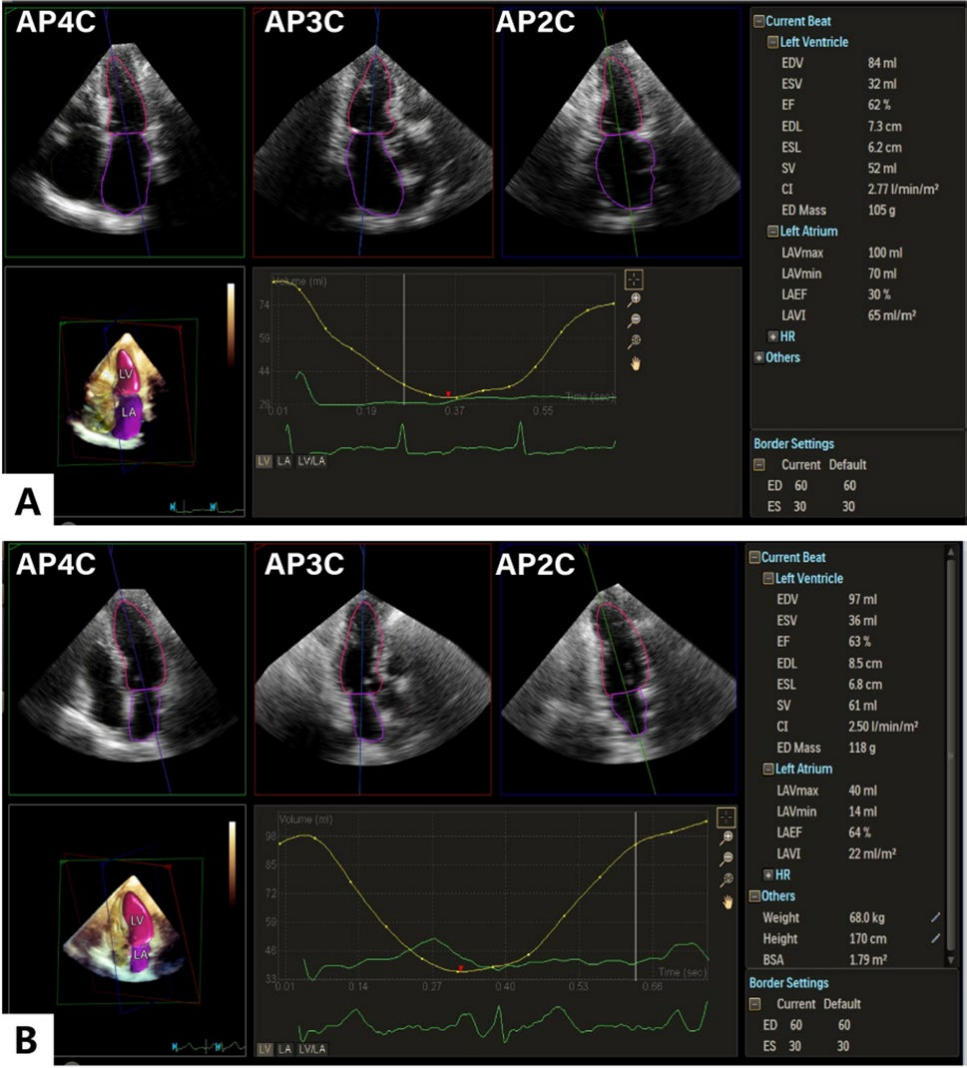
Left atrial ventricular coupling index (LACI). Using three-dimensional echocardiography and AI intelligent quantitative software Dynamic Heart Model (DHM) automatically obtained the new echocardiographic parameter left atrial ventricular coupling index (LACI). A, B 2D apical four-, three-, and two-chamber views separately extracted from coronary artery diseases (CAD) patients with heart failure and without major adverse cardiac event (MACE) at end-diastole. Red and purple lines denote LV and LA endocardial borders, respectively, registered using fully automated software. AP2(3,4)C, apical two-(three-, four-)chamber view; CI, cardiac index; ED(S)L, end-diastolic (end-systolic) length; ed(es)LVLAVR, left ventricular-left atrial volume ratio at LV end-diastole (end-systole); ED(S)V, end-diastolic (end-systolic) volume; EF, ejection fraction; LA, left atrium; LAEF, left atrial ejection fraction; LAVI, left atrial volume index; LAVmax, maximal left atrial volume; LAVmin, minimal left atrial volume; LV, left ventricular; LVEDV, left ventricular end-diastolic volume; LVEF, left ventricular ejection fraction; LVESV, left ventricular end-systolic volume; SV, stroke volume
